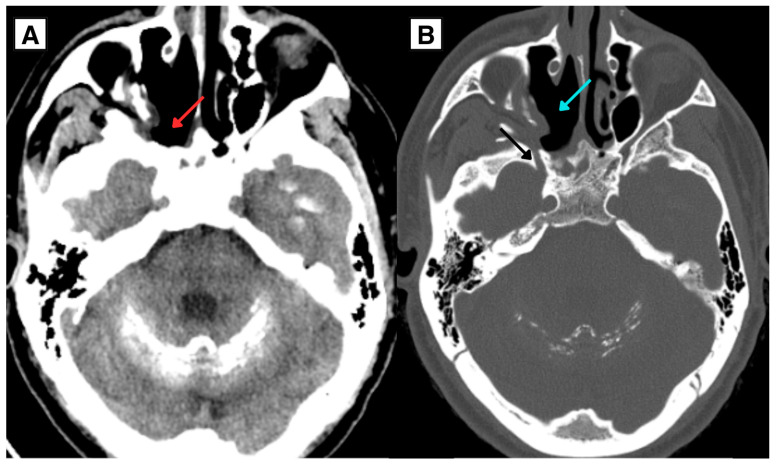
Follow-up non-contrast CT of the head/paranasal sinuses 3 months after endoscopic resection of the right-sided nasopharyngeal mass. (A) Axial CT demonstrates postoperative changes without evidence of residual or recurrent mass (red arrow). (B) Axial CT demonstrates postoperative bony defects in the medial wall of the maxillary sinus (blue arrow) and enlarged foramen rotundum (black arrow). Extensive intracranial calcifications persist.
